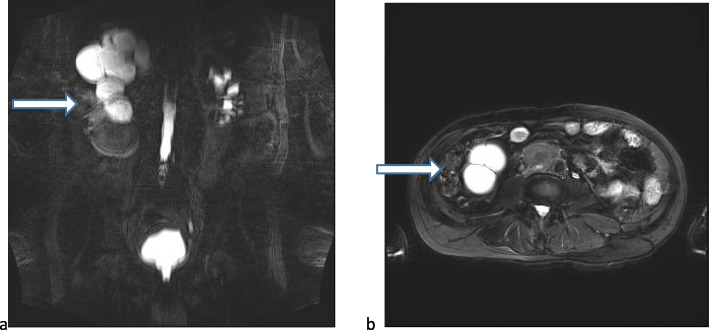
Coronal magnetic resonance imaging (MRI) (a) and axial MRI (b) showing huge hydronephrosis of the right kidney with plaque-like abnormal signal in the middle portion (malignant mass). *White arrow* shows the mass
